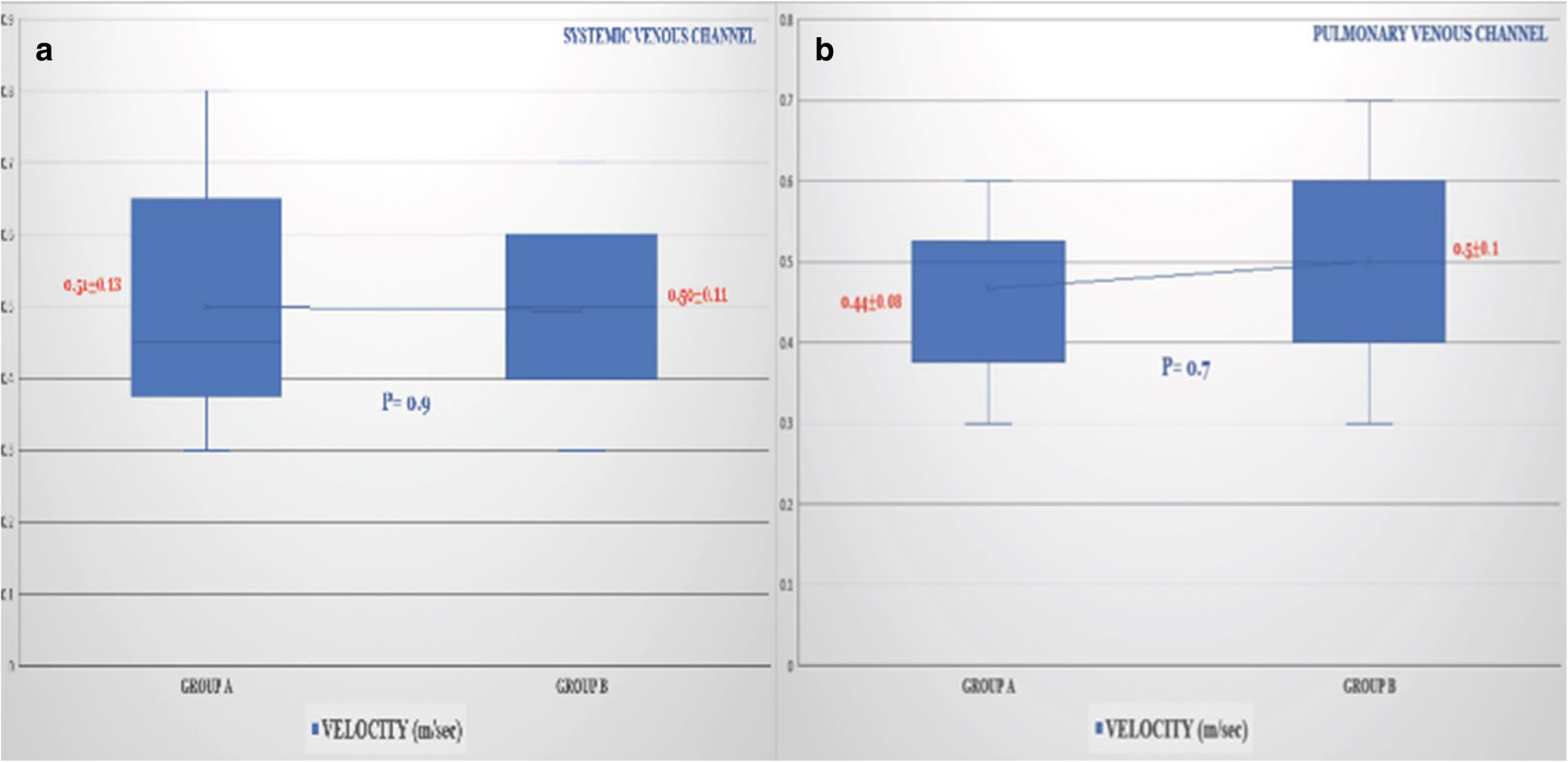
Velocity by echocardiographic doppler assessment of a systemic and b pulmonary venous channels
